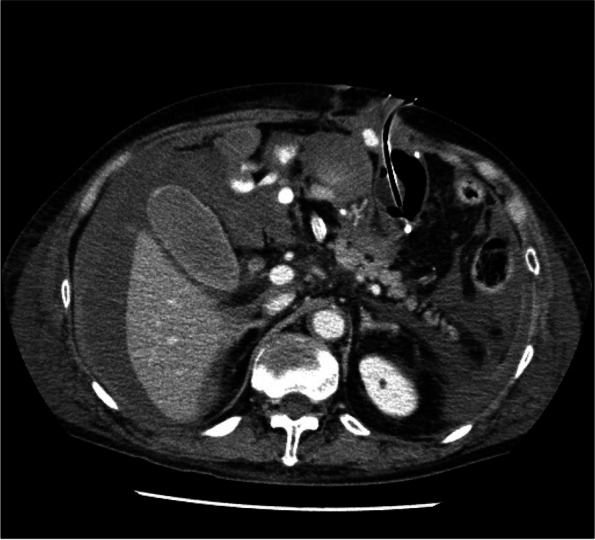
CT on the 16th POD revealed a large amount of bloody ascites due to active bleeding from the tube jejunostomy site
